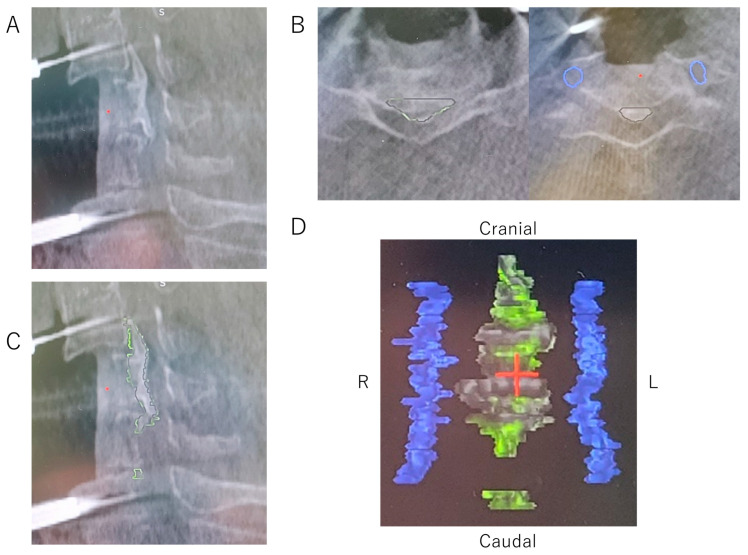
Intraoperative CT images of the OPLL. (A) Intraoperative CT sagittal view of the OPLL. (B) Outline of ossification (yellow-green line) and blood vessels (blue line) marked on the monitor of the CT axial views. (C) Sagittal image of the OPLL, which was reconstructed after marking the outlines of ossification for each axial slice from the cranial end to the caudal end of the OPLL. (D) Reconstructed 3D images of the OPLL and bilateral vertebral arteries. CT, computed tomography; OPLL, ossification of the posterior longitudinal ligament.
